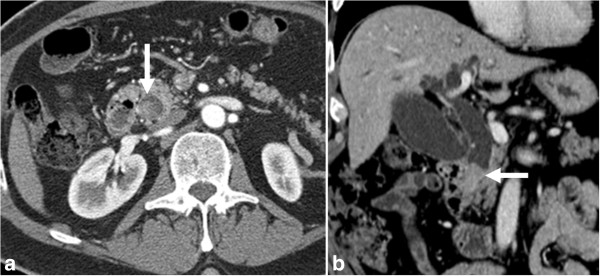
Distal CCA presenting as a polypoid mass with obstructive jaundice. Contrast enhanced CT axial (a) and coronal reconstruction (b) showing a soft tissue density filling defect in distal common bile duct (arrow) representing the invasive grade 3 adenocarcinoma.
